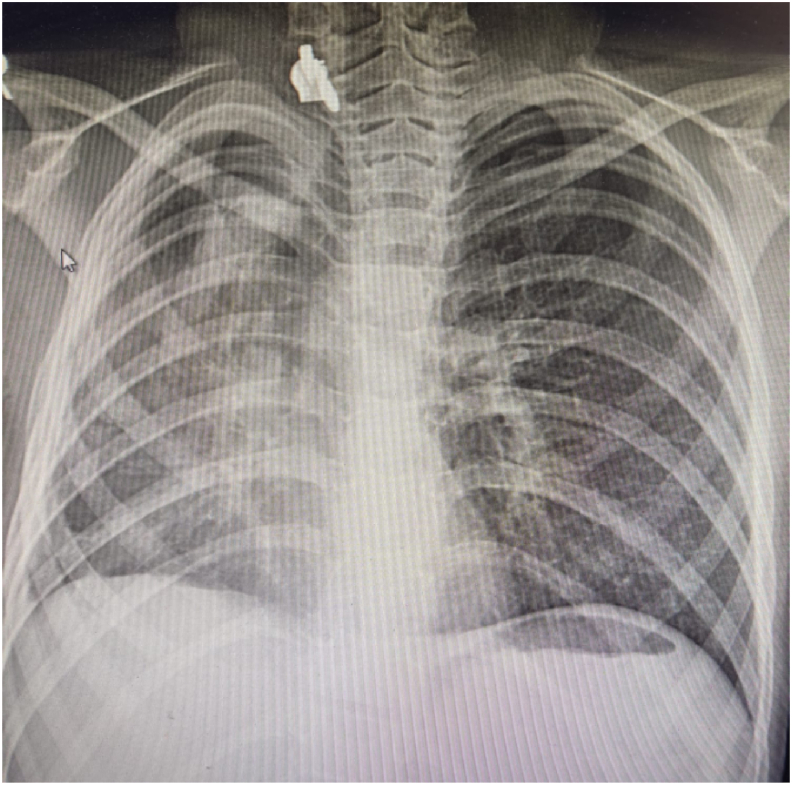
Chest radiography after stent removal which shows full expansion of the right lung.
